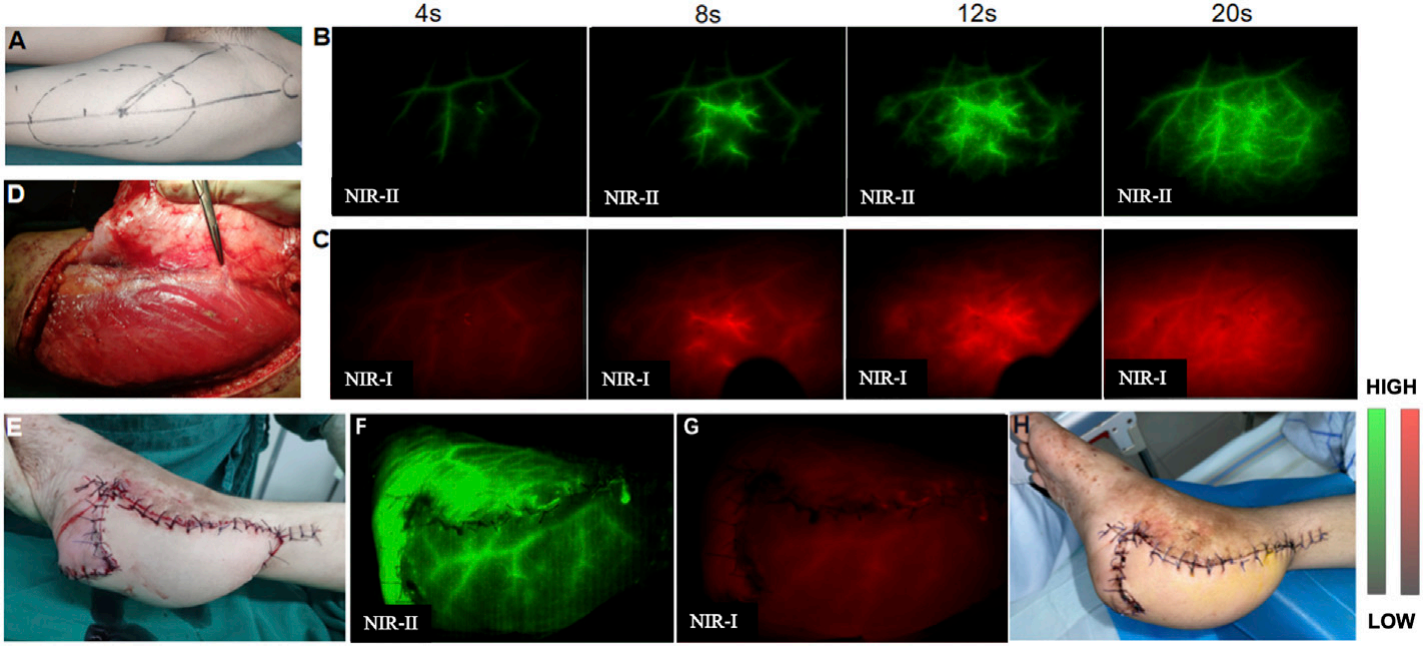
A 18-year-old man with the left heel scar contracture who underwent scar excision and reconstructive surgery by microsurgical free anterolateral thigh flap transfer. (A) One perforator was preliminarily located by Doppler sonography before performing NIR imaging. (B,C) Preoperative NIR-II imaging revealed four possible perforators, one of which overlapped with the Doppler location. (D) Perforators were found intraoperatively, and their locations agreed exactly with those observed by NIR imaging. (E) Flap transplantation was completed. (F,G) NIR-II imaging displayed satisfactory flap perfusion and clearer revascularization than NIR-I imaging after transplantation. (H) The flap showed complete viability and there were no complications 7 days postoperatively.
